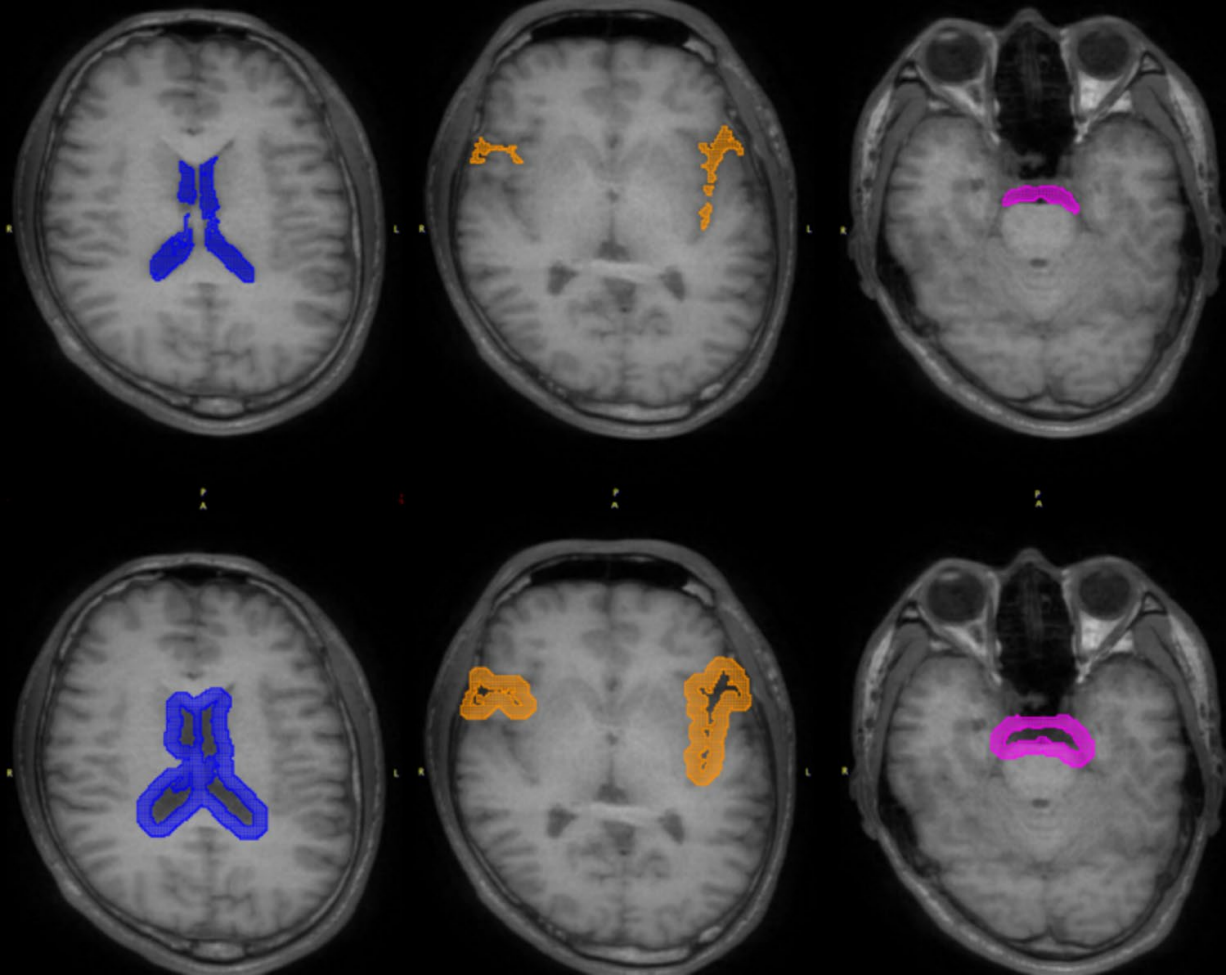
Examples of VOI in the CSF region and surrounding areas. VOIs for the CSF regions (upper row) and their corresponding surrounding regions (lower row) are shown on MRI-T1 images from a healthy individual. The LV is indicated in blue, the FS in orange, and the PPC in pink
